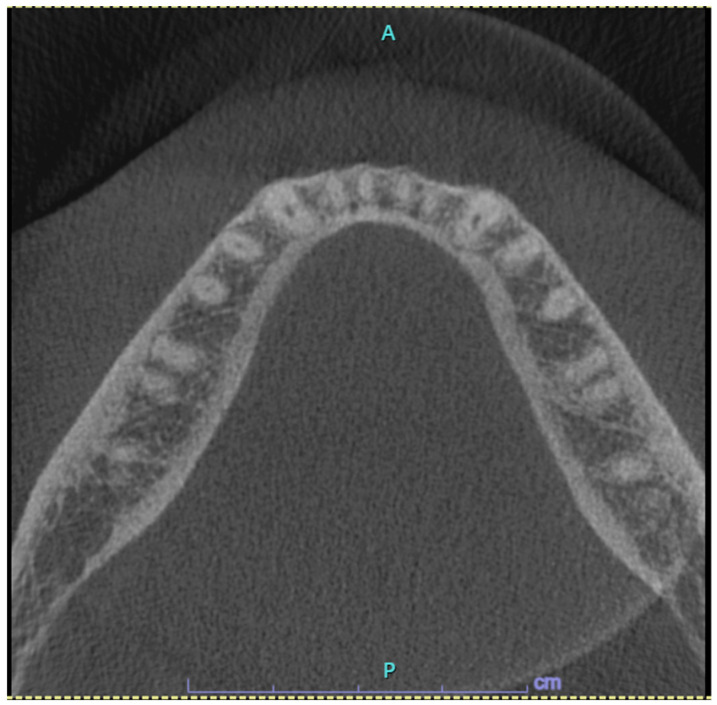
Axial Cone beam computed tomography (CBCT) sections showed the absence of altered density of trabecular bone, radiolucency, bone expansion, or changes in the mandibular lingual cortical bone in the right premolar area.
